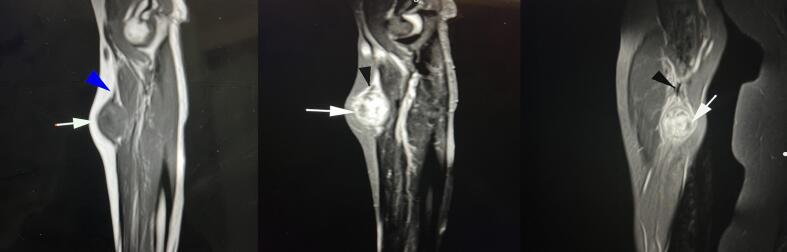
Sagittal T1 (a), sagittal PD fat saturation(b), and coronal PD fat saturation (c) MR images show well-defined soft tissue mass which is isosignal in T1 and high signal in T2. it shows Some T1 high signal components. Note also hypo signal components due to turbulent flow in all images (arrows). signal void radial artery (arrowheads)could also be visible, reaching this partially thrombosed aneurysm.
